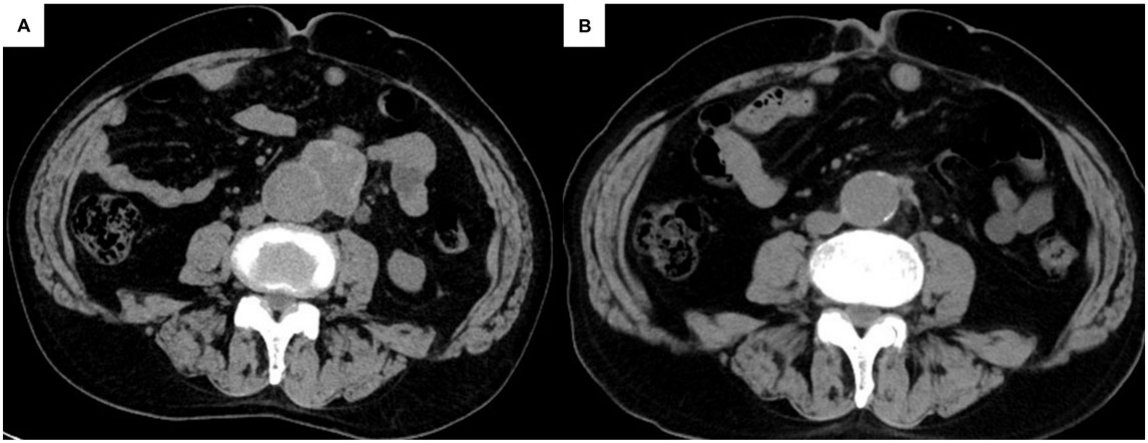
(A) Preoperative computed tomography image showing pseudoaneurysm of the abdominal aorta. (B) Postoperative computed tomography image showing repaired abdominal aorta without aneurysmal change.
